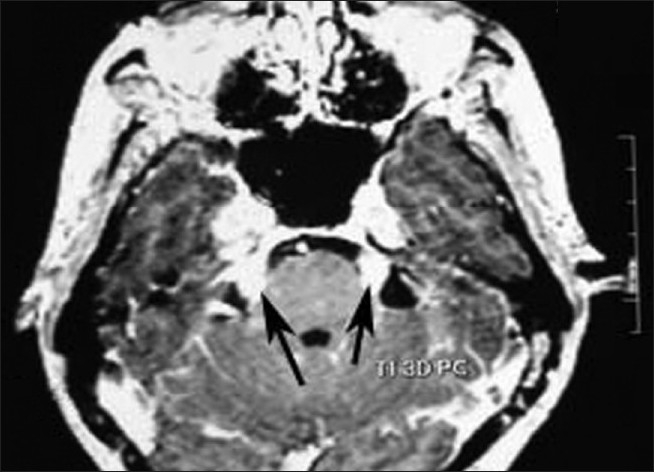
T1-weighted post-contrast images showing thickened and enhancing trigeminal nerves bilaterally as shown by arrows
